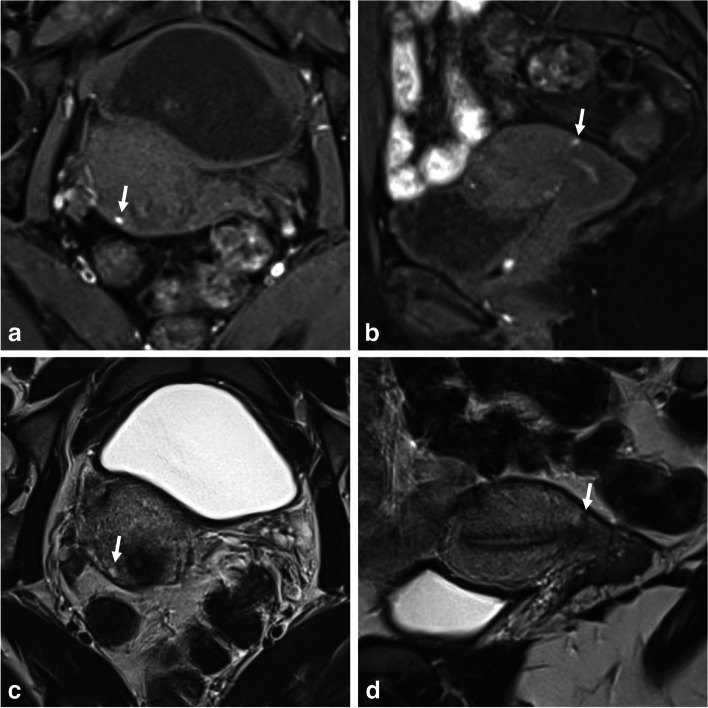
Flow-related enhancement artifact, also known as “the entry slice phenomenon”, in a 36-year-old woman with chronic pelvis pain and dysmenorrhea. a Axial and (b) sagittal reconstruction T1-W fat-suppressed MR image show a bright spot (arrows) in close proximity with the right USL insertion, also present on contiguous slices (not shown). c Axial and (d) sagittal T2-W MR images show a sub-serous vein (arrows) in place of the T1 bright spot, near the right USL insertion
